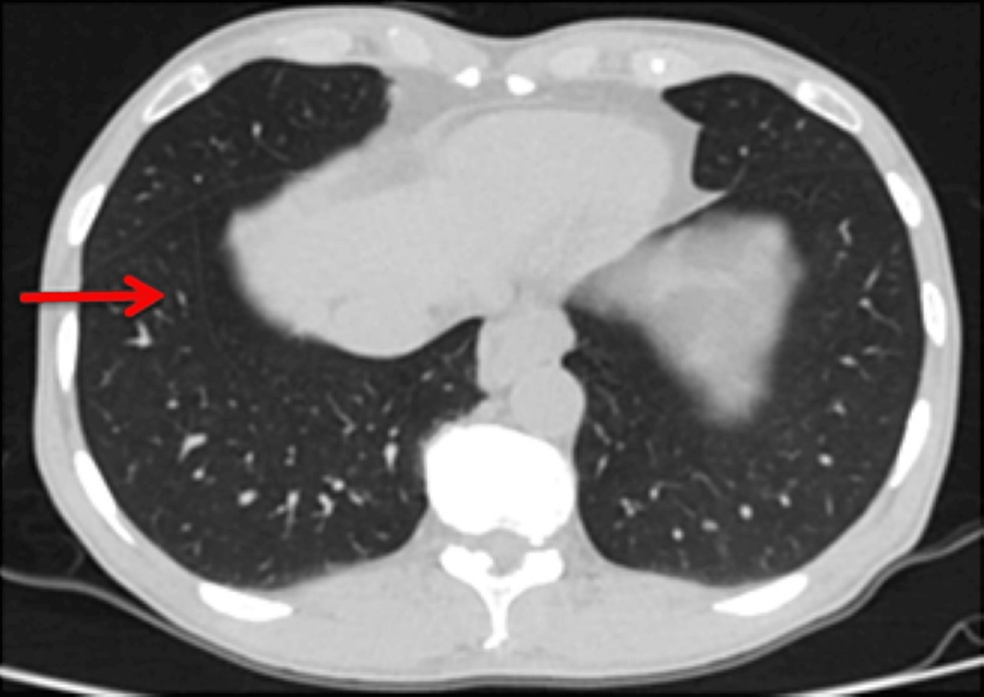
High-resolution computed tomography (HRCT) chest axial image shows slender curvy-linear white line (red arrow) separating the medial basal segment of the right lower lobe from the remaining segments, the inferior accessory fissure
